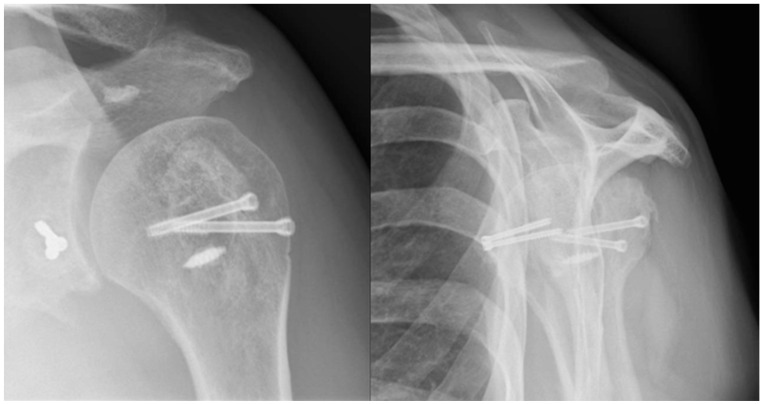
Follow-up radiographs. Anteroposterior and lateral “Y-view” radiographs at 24 months showing no change in the reduction, and no signs of complications.
